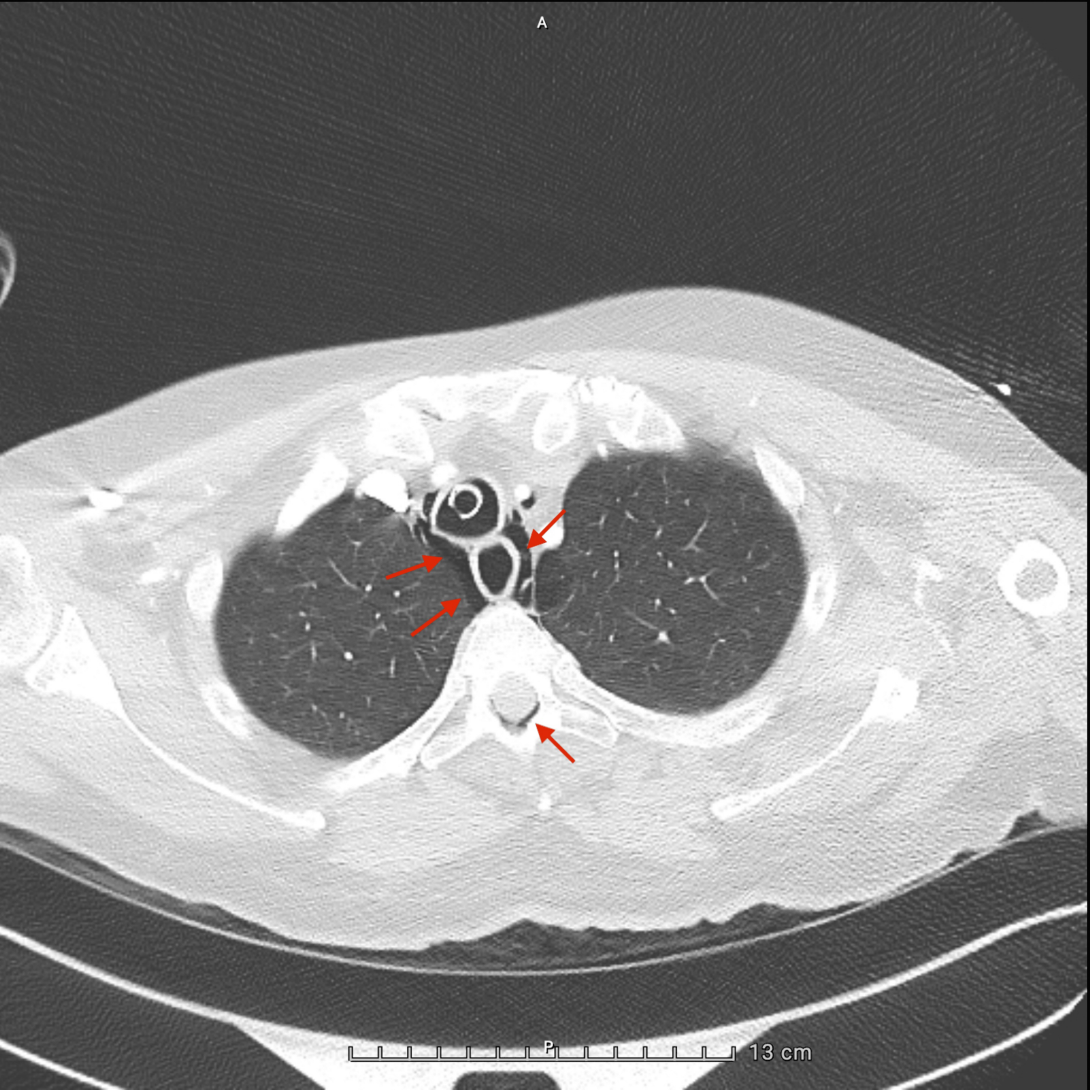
Axial chest CT – upper thoracic level slice showing pneumomediastinum and epidural air.
